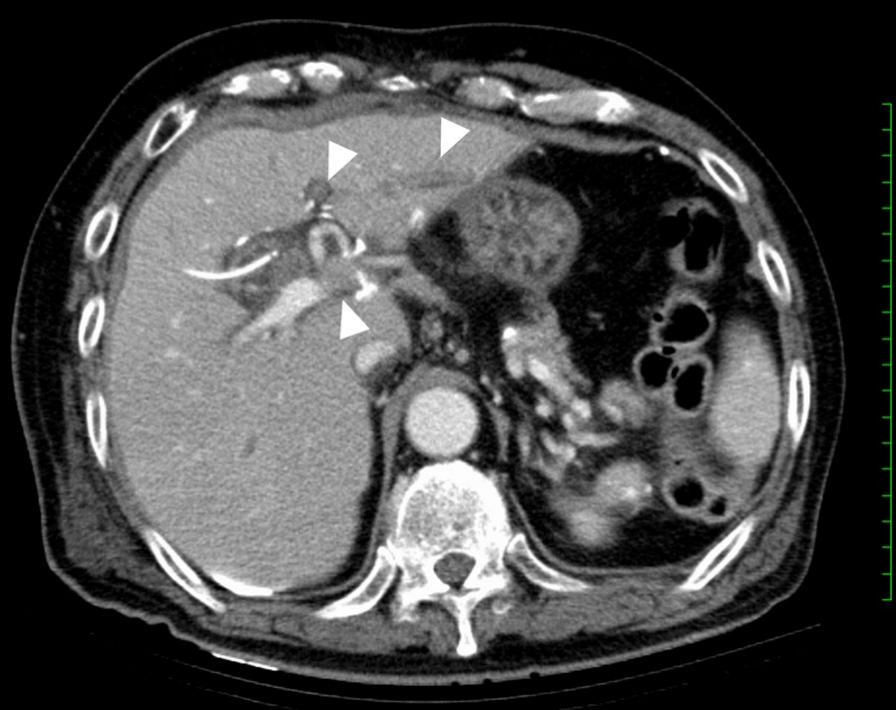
Abdominal computed tomography (CT) images of case 1. An enhanced abdominal CT scan showed thrombosis in the left and anterior segment branches of the portal vein (white triangle) on POD 1
